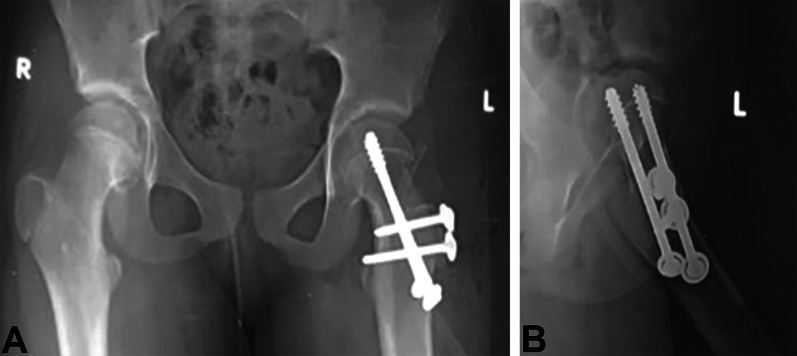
Immediately postoperative X-rays. A AP view. B Lateral view
